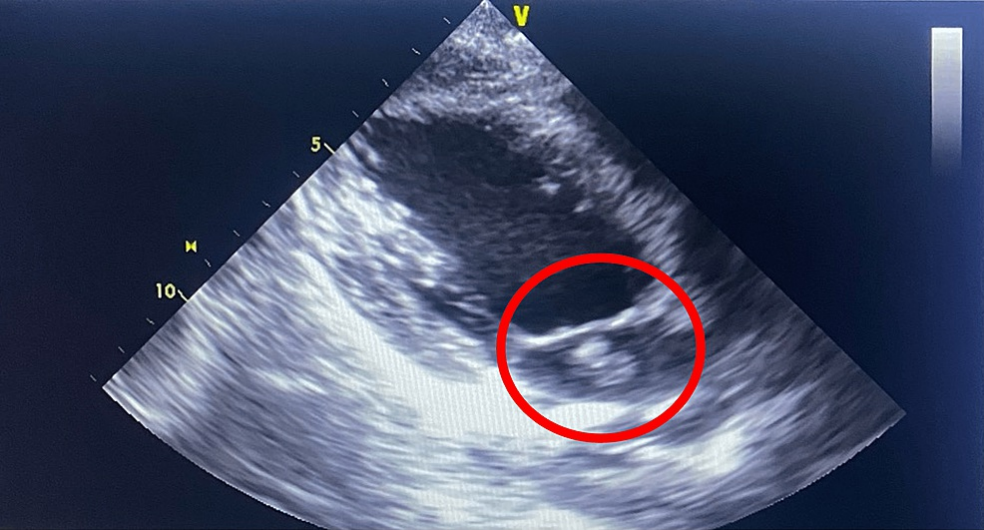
Transthoracic echocardiography indicating large vegetation near the base of the posterior mitral annulus on the left atrial side (indicated in the red circle)
